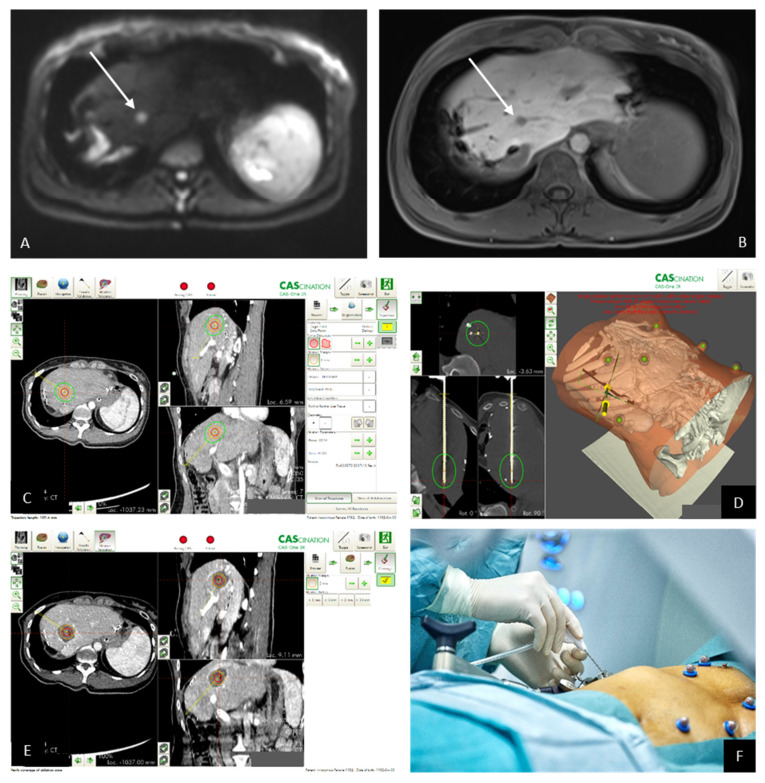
(A) Pre-interventional MRI imaging reveals the metastasis with high signal in the b800 image of the diffusion-weighted imaging (DWI) and also shows (B) a lack of intracellular uptake of hepatocyte-specific contrast medium in the hepatobiliary phase. (C) The pre-interventional planning of the ablation zone (red circle = segmented tumor; (D) green circle = anticipated ablation zone) needle validation scan with the needle in place and the anticipated ablation zone in green. (E) The ablation zone in the contrast-enhanced control scan; (F) needle in place.
